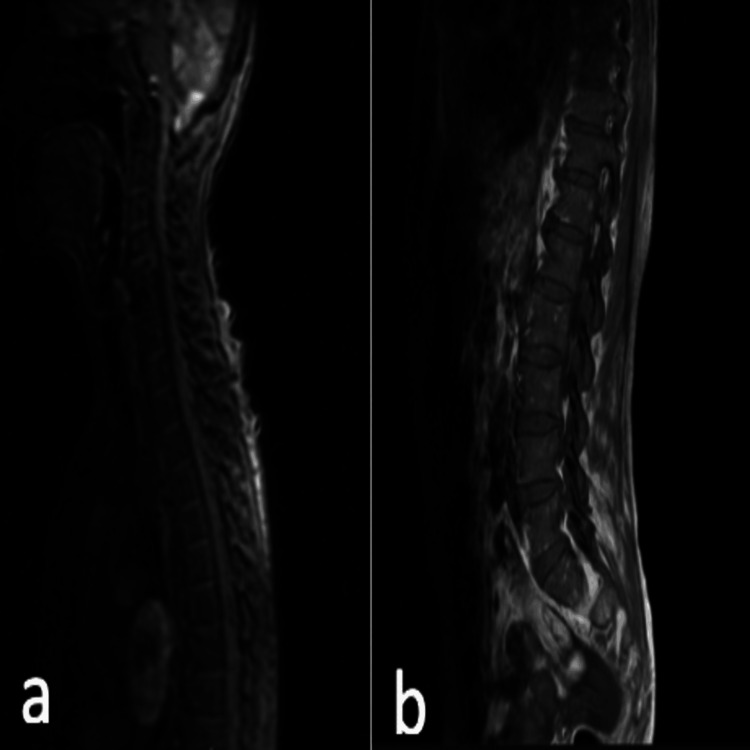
T1 and sagittal MRI spine showing multilevel degenerative spondylotic changes in the cervical and lumbar spine. (a) Cervical MRI and (b) lumbar MRI.
